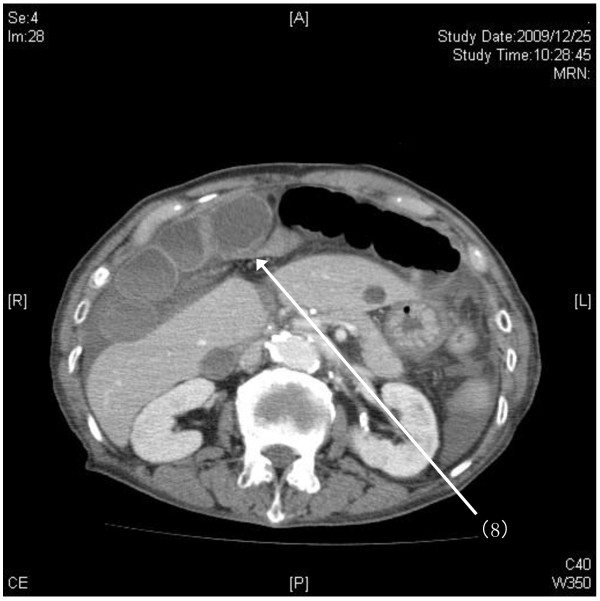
Pre-operative abdominal helical computed tomography (CT): horizontal view 2. Another cephalic horizontal view showed the strangulated distal intestine through a hilar defect (8) in the falciform ligament.
